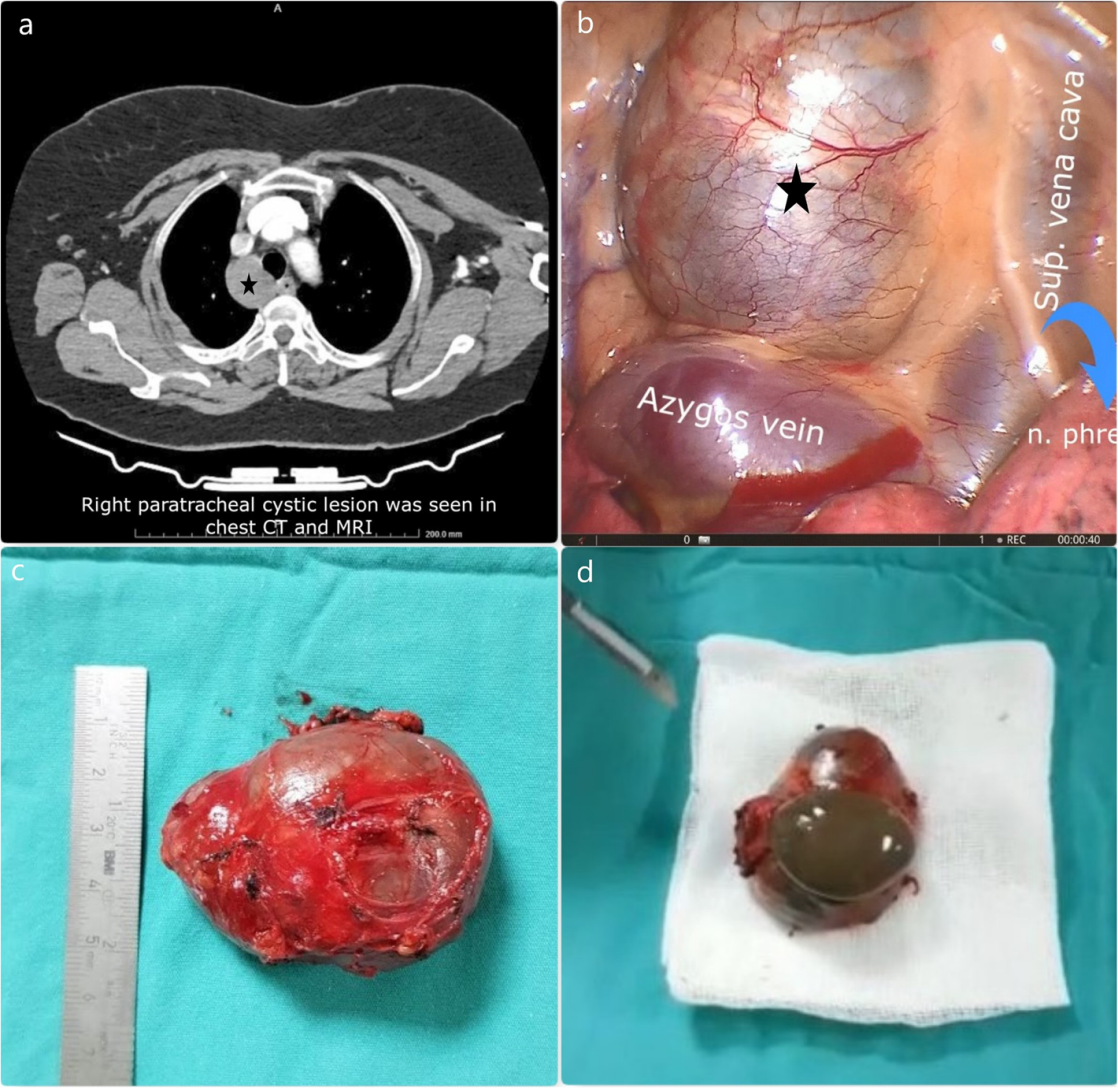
The right mediastinal cystic lesion was observed in the thorax CT (Fig. 1a). VATS resection of the mediastinal bronchogenic cyst (Asterisk) was performed. Brown mucoid content was observed in the macroscopy of the resection material (Fig. 1d)
